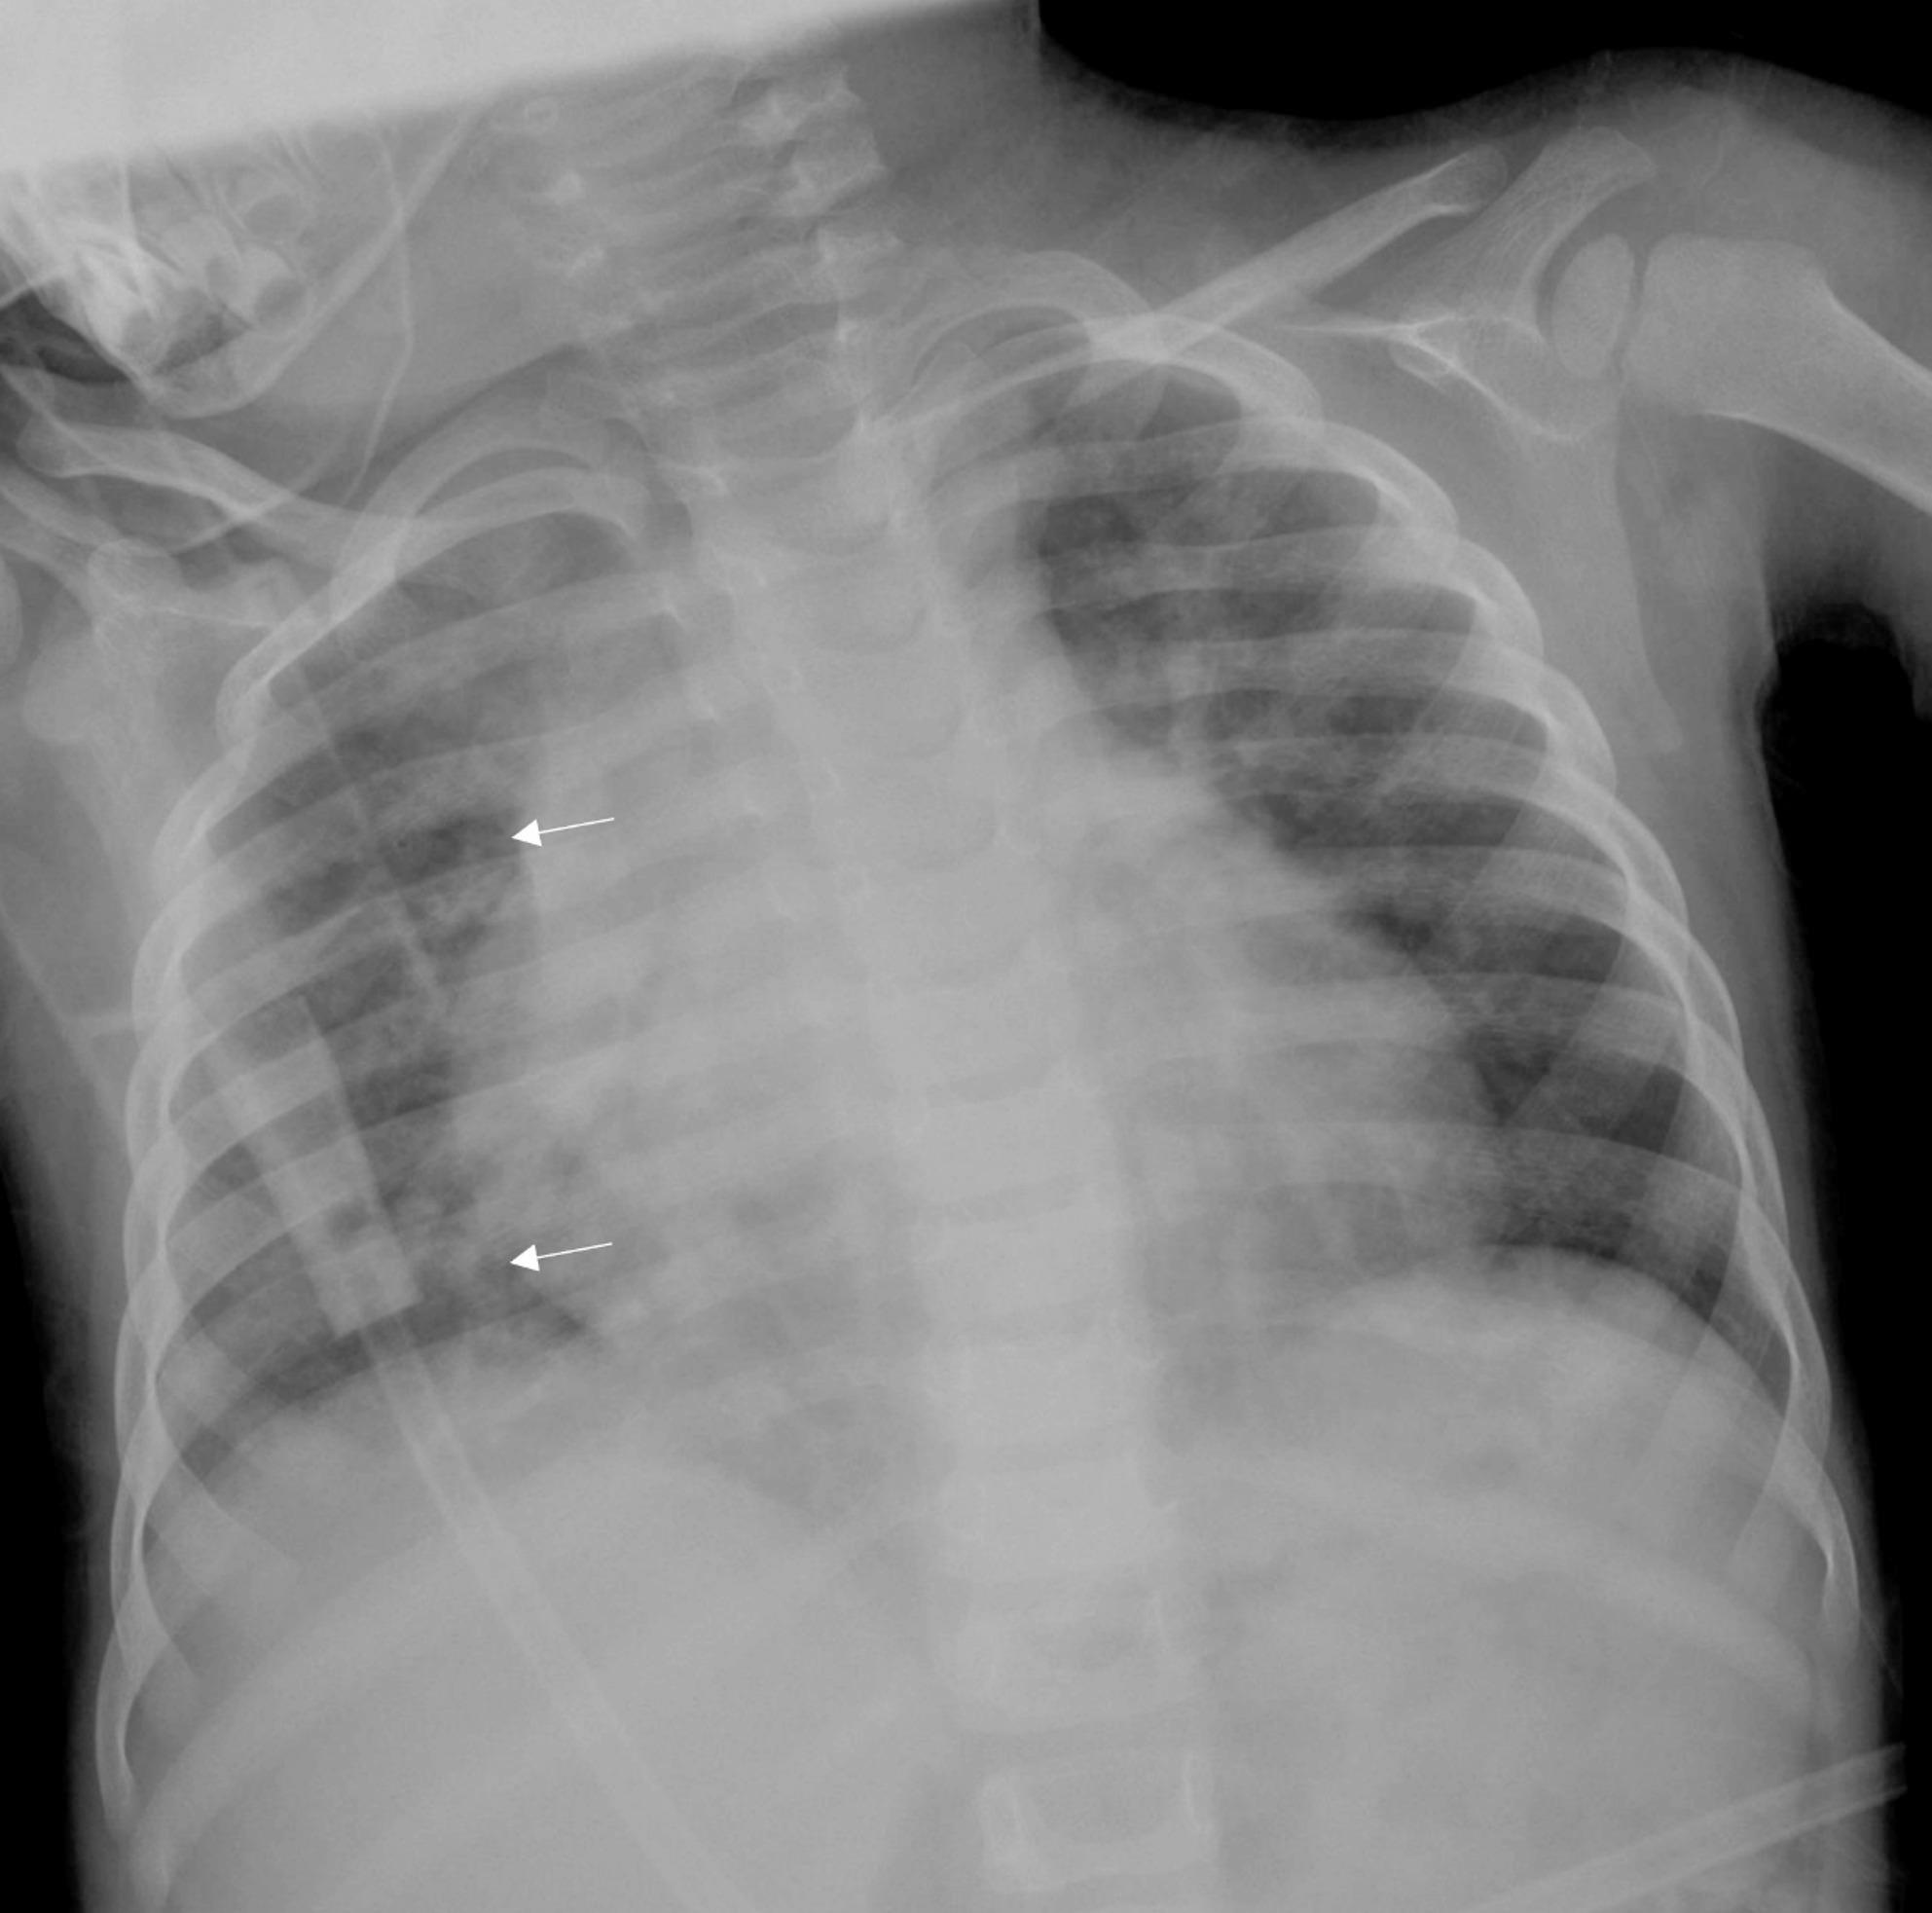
Chest X-ray demonstrating diffuse patchy pulmonary infiltrates (arrows)
